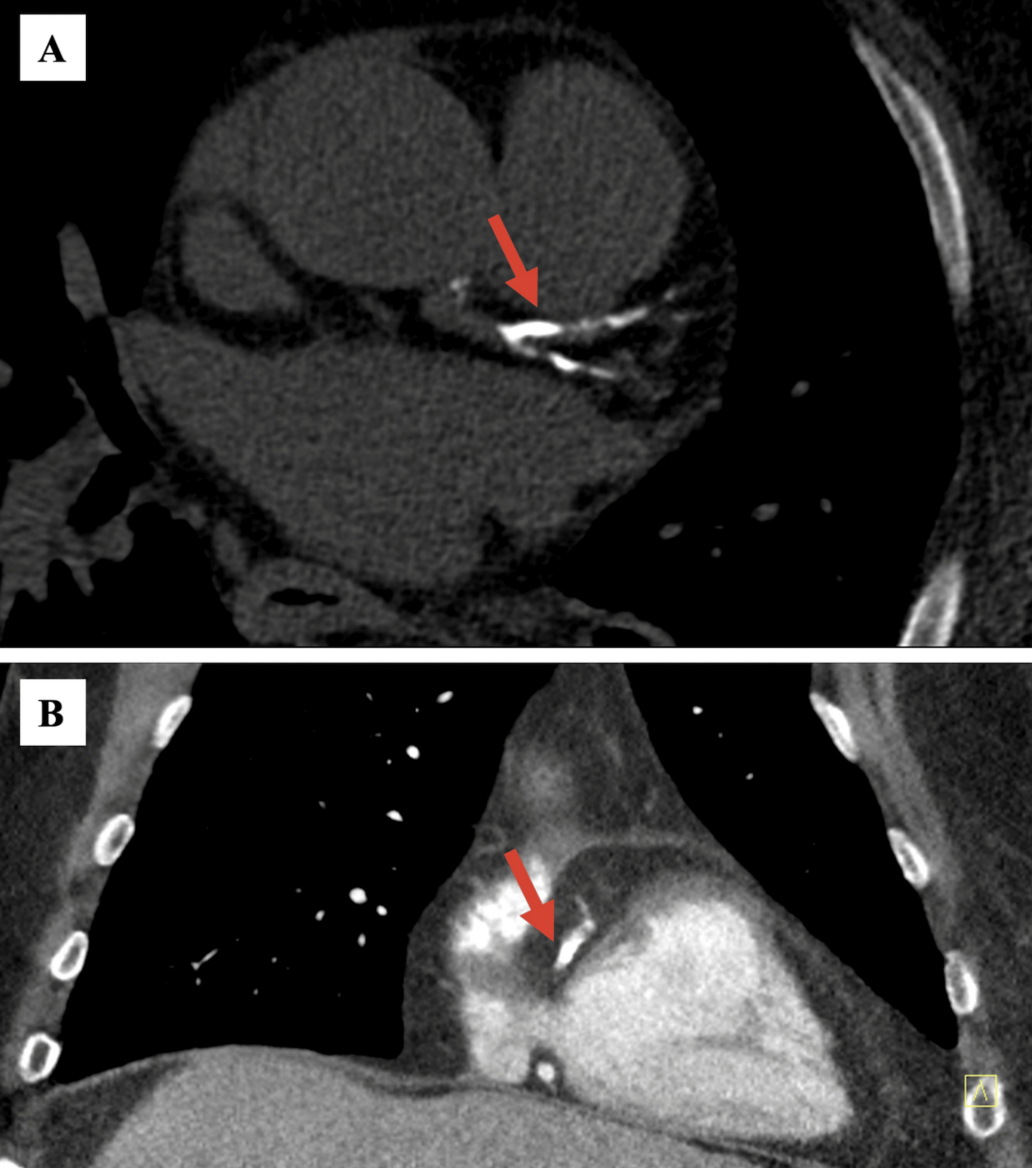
Computed tomography angiogram (CTA) results. Contrast-enhanced CTA images of severe (A) left anterior descending artery calcifications in the transverse plane and (B) right coronary artery calcifications in the coronal plane (red arrows), prompting suspicion for flow-limiting stenoses.
